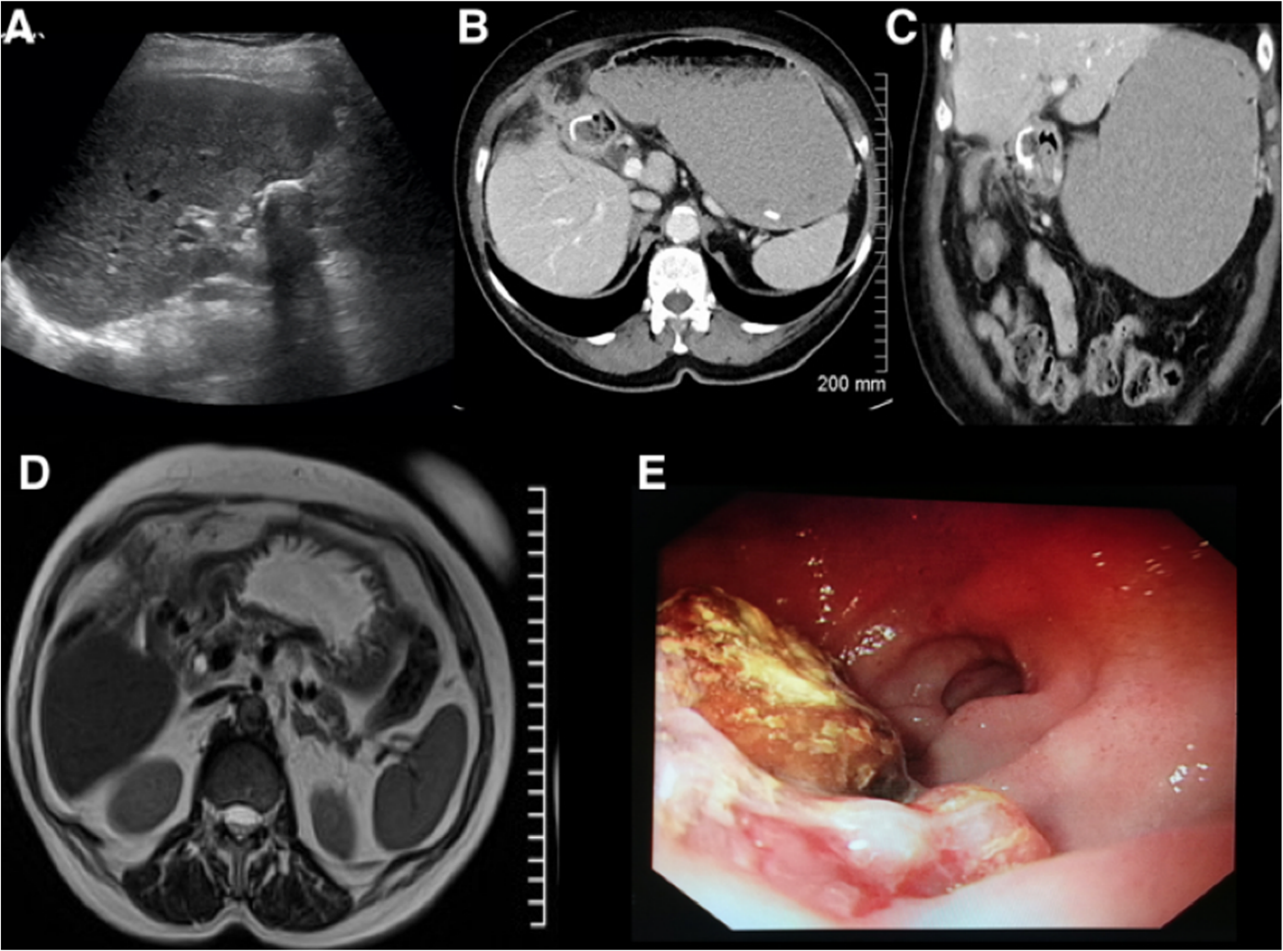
Ultrasound (a), CT (b, c), MRI (d) and endoscopic images (e). Demonstrating a large calcified gallstone in the proximal duodenum with a massively dilated stomach. Findings are consistent with a proximal bowel obstruction consistent with Bouveret’s syndrome
